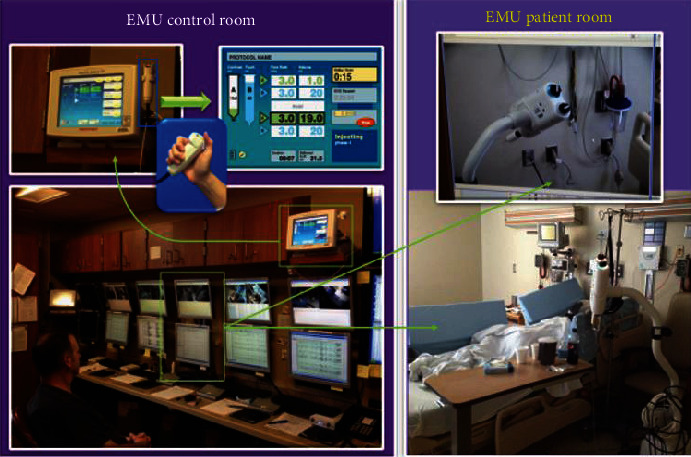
Demonstration of the use of automatic SPECT injectors at the Epilepsy Monitoring Unit (EMU) of the University of Pittsburgh Medical Center (UPMC) at Presbyterian University Hospital (PUH). Automatic injectors are armed by nuclear medicine staff. The radiotracer is injected upon a button press by the EMU staff in the EMU control room (pictures on the left side) upon observing clinical or electrographic seizure affecting the monitored patient (pictures on the right side).
